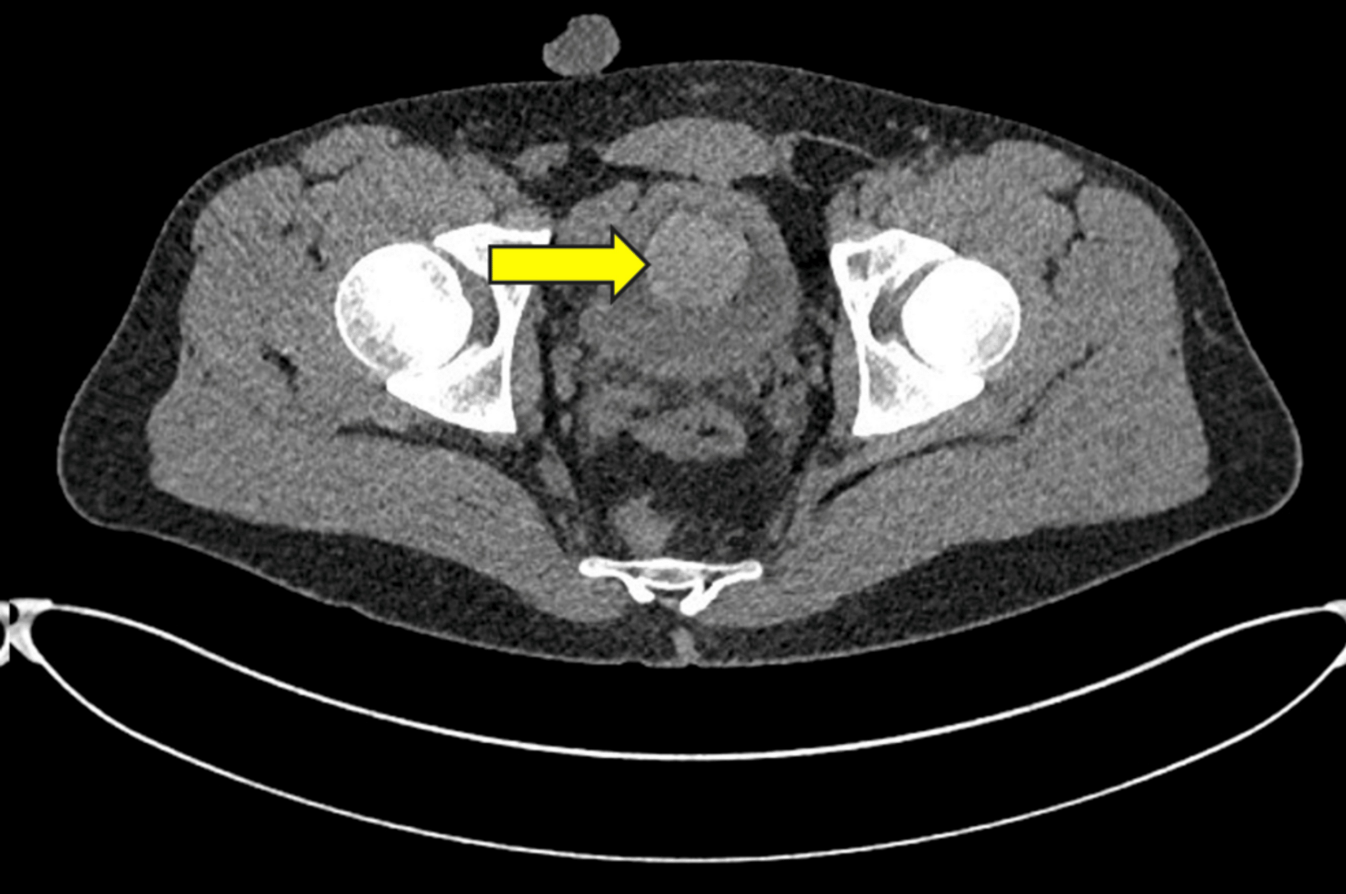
Axial CT image demonstrating a radio-opaque silicone material collected within the bladder (yellow arrow).
